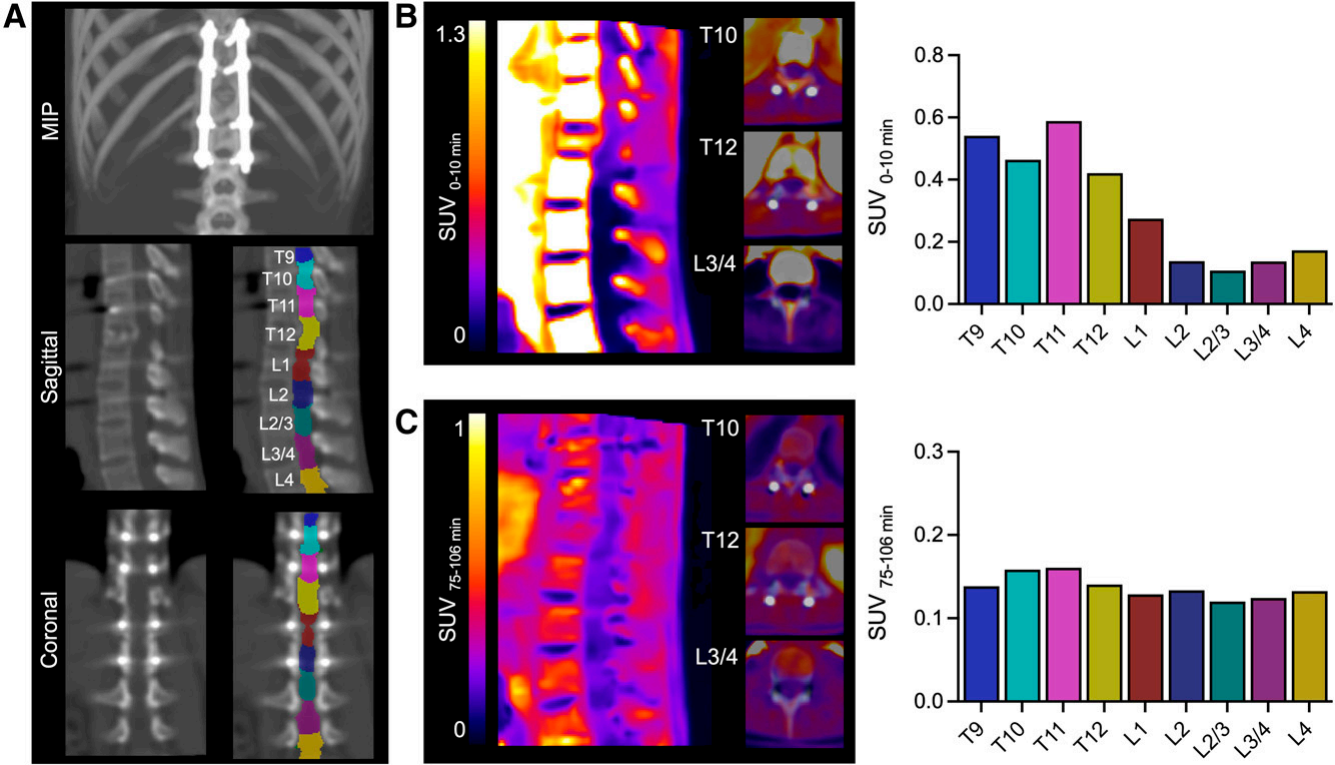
[^18^F]3F4AP in a human subject after severe incomplete SCI. (A) Maximum-intensity projection (MIP), sagittal, and coronal CT images showing location of metal stabilization hardware and crushed vertebral body at T12. Selected regions of interest for segmentation of spinal cord are shown for both sagittal and coronal views. (B) Early PET sagittal images showing compressed vertebral body and low PET signal in cord below injury and quantification 0–10-min SUV at selected spinal segments. (C) Late PET sagittal images showing reduced PET signal in cord below injury and quantification of 75–106-min SUV at selected spinal segments. For both early and late PET, axial images at T10, T12, and L3/4 are shown.
